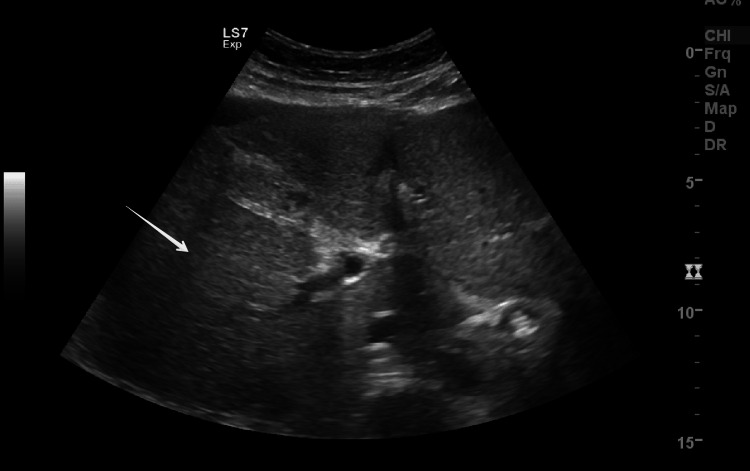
Ultrasound image shows increased echogenicity with fatty infiltration
